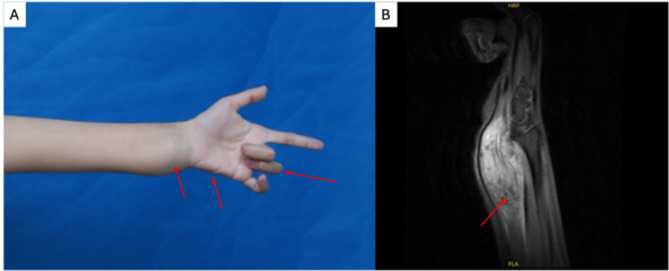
Clinical manifestation and Magnetic Resonance Imaging (MRI) of the lump at the wrist. A: Ulnar nerve entrapment: D3 and D4 extending limitation and hypothenar atrophy (the arrows). B: Flowing void effect in the lesion of T2 (the arrow)
